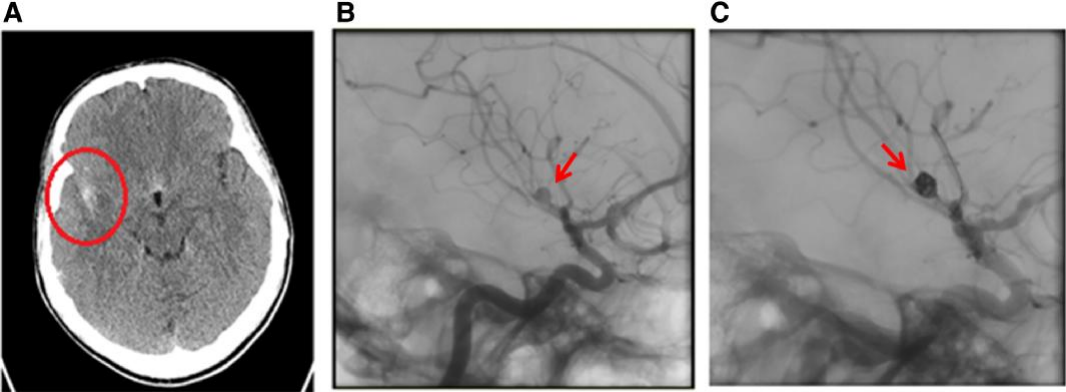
(*A*) Computed tomography brain shows acute subarachnoid haemorrhage with blood mainly seen in the right Sylvian fissure. (*B*) A 4 mm irregular surface aneurysm (arrow) with wide neck incorporating the segment of the artery arising from the proximal trunk of the superior M2 bifurcation of the right middle cerebral artery. This appears to be an acutely ruptured dissecting aneurysm. (*C*) The mycotic aneurysm post-coiling (arrow).
